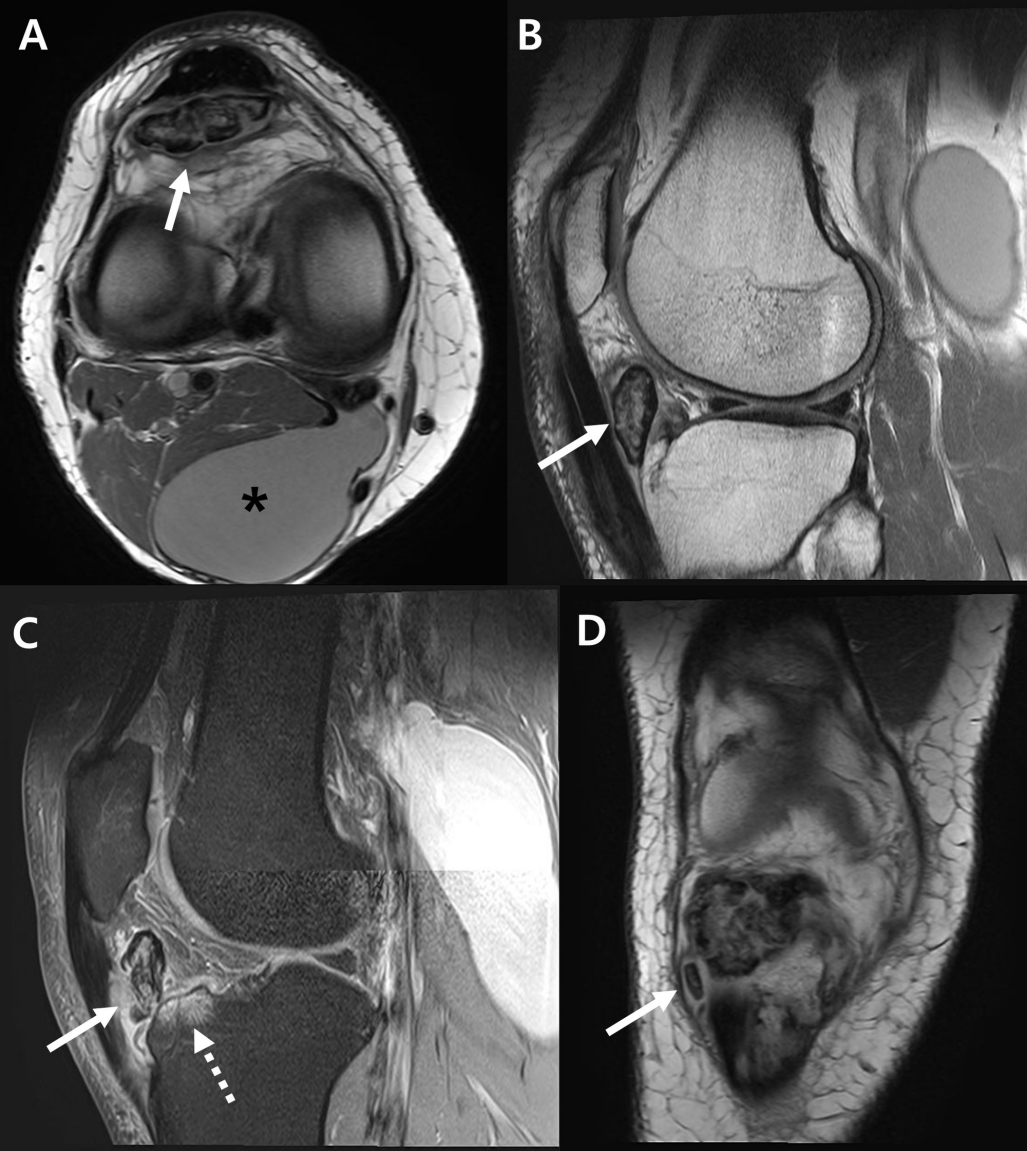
Right knee magnetic resonance images. (A) A large ossicle with heterogenous signal intensity (arrow) and a Baker’s cyst (asterisk) are seen on axial image. (B) From T1-weighted sagittal image, the ossicle (arrow) is separately located inside the infrapatellar fat pad. (C) T2-weighted sagittal image shows inflammation of patellar tendon (solid arrow) and bone marrow edematous change of anterior tibia plateau (dotted arrow). (D) Small separated ossicle (arrow) was found inferior to the large ossicle from coronal image.
